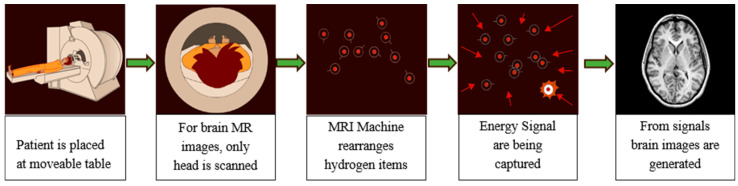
MR image capturing process from MRI Scanner.
